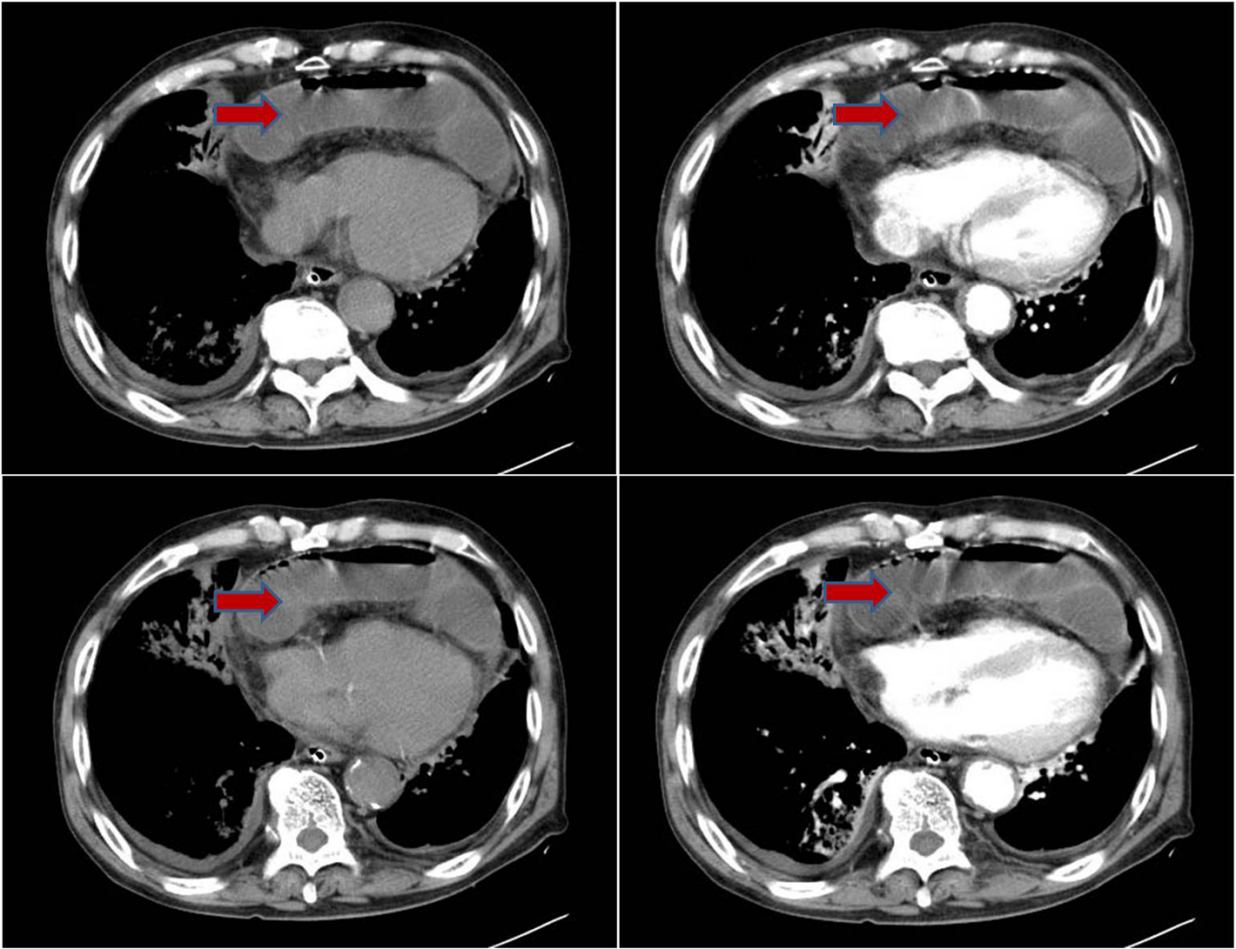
Computed tomography (CT) demonstrates a loop of intestine in the pericardial space (arrow).
